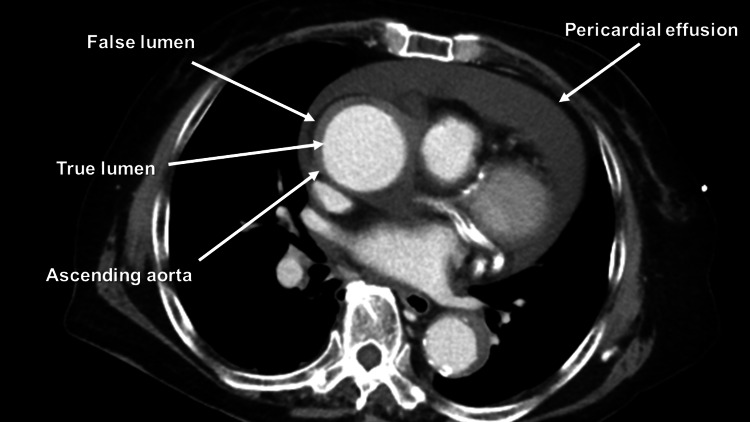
Preoperative contrast-enhanced computed tomography Preoperative contrast-enhanced computed tomography revealing type A acute aortic dissection and pericardial effusion
